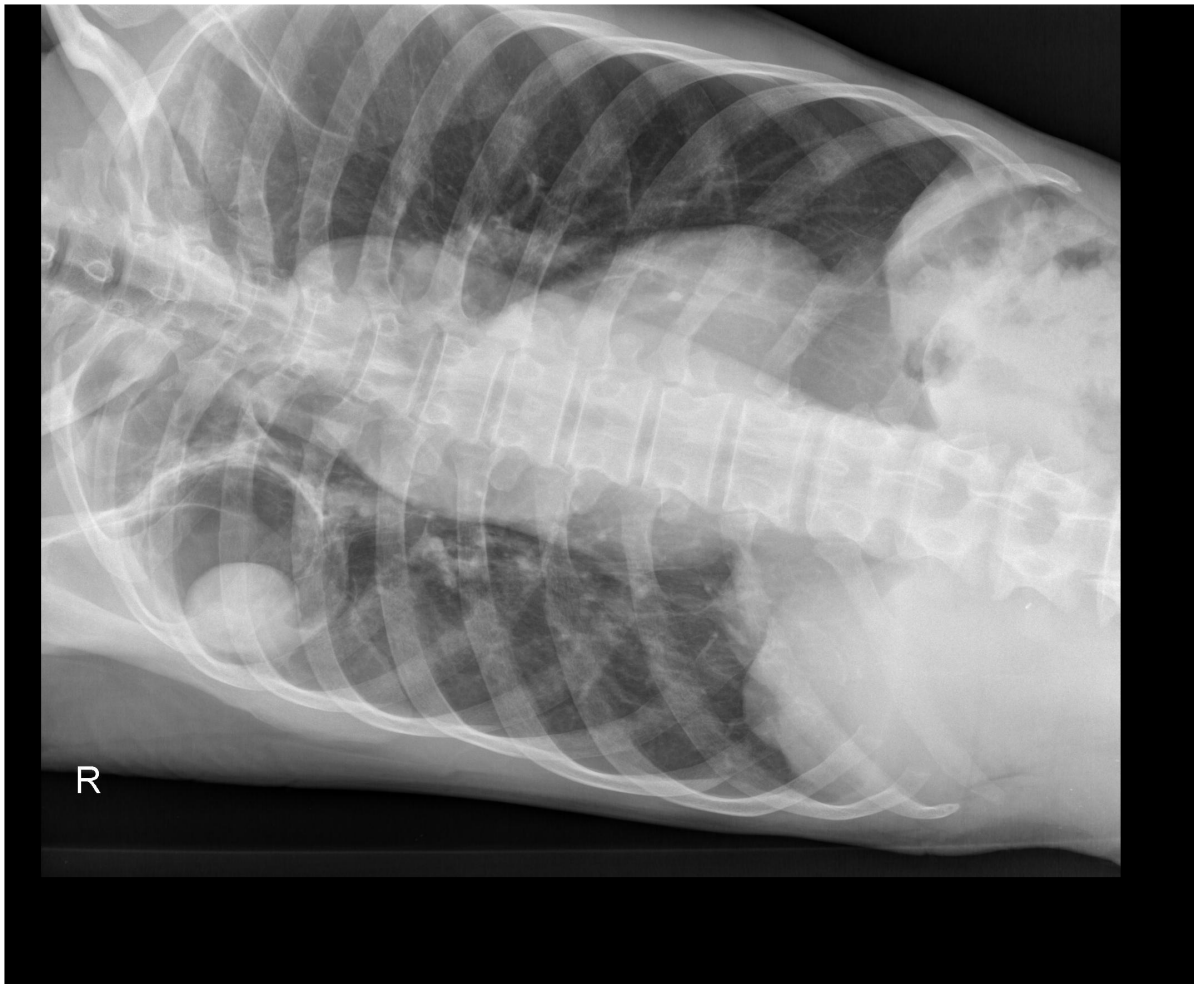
Chest radiography in supine position showing a change of position of the fungus ball.
